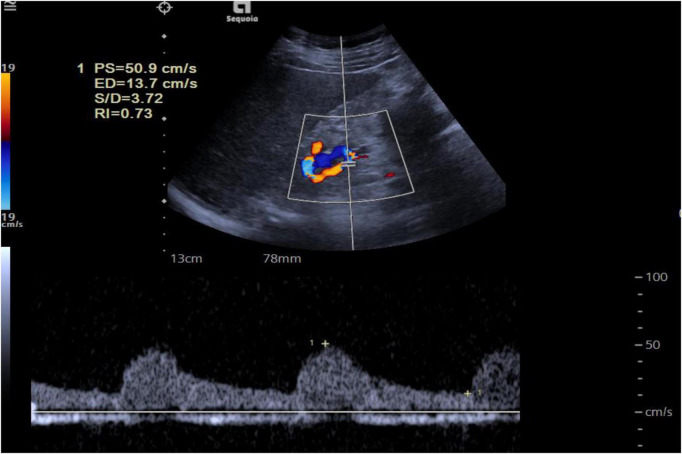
Representative sonographic image of stage 2 CKD and arterial blood flow.
